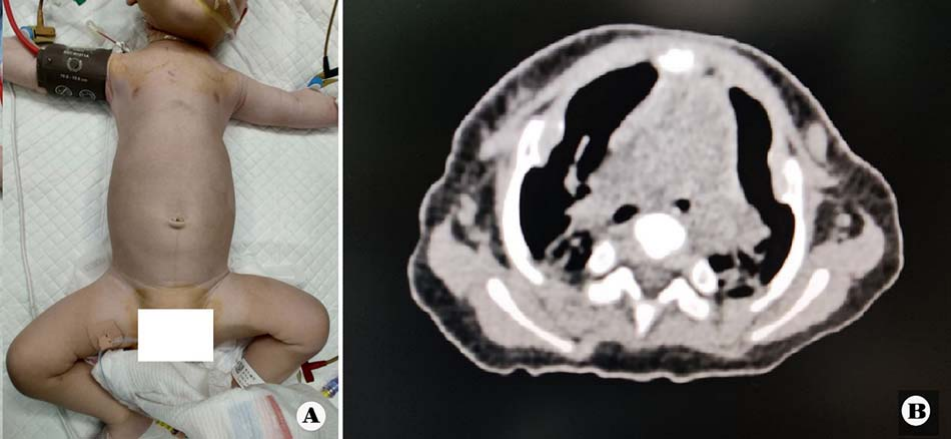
(A) Thorax appearance before operation, and (B) CT scan image of thorax before operation.
